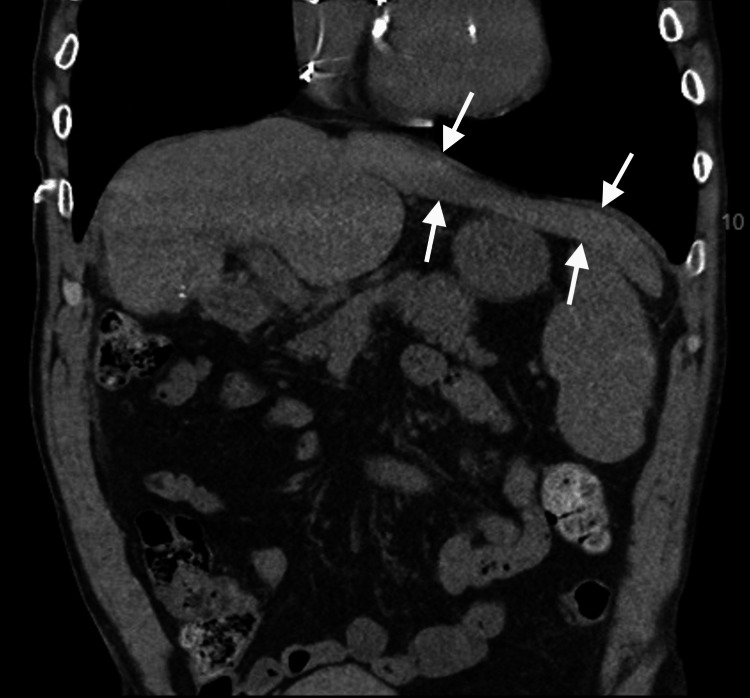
CT of the abdomen shows the incidental finding of the beaver tail liver as indicated by the arrows.
